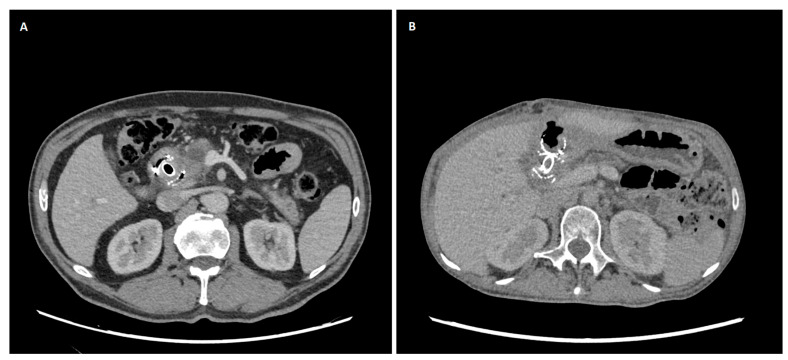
Computed tomography (CT) scans of patients who underwent endoscopic ultrasound-guided biliary drainage due to malignant biliary obstruction (MBO). (A) Cholecystogastrostomy. (B) Choledochoduodenostomy.
